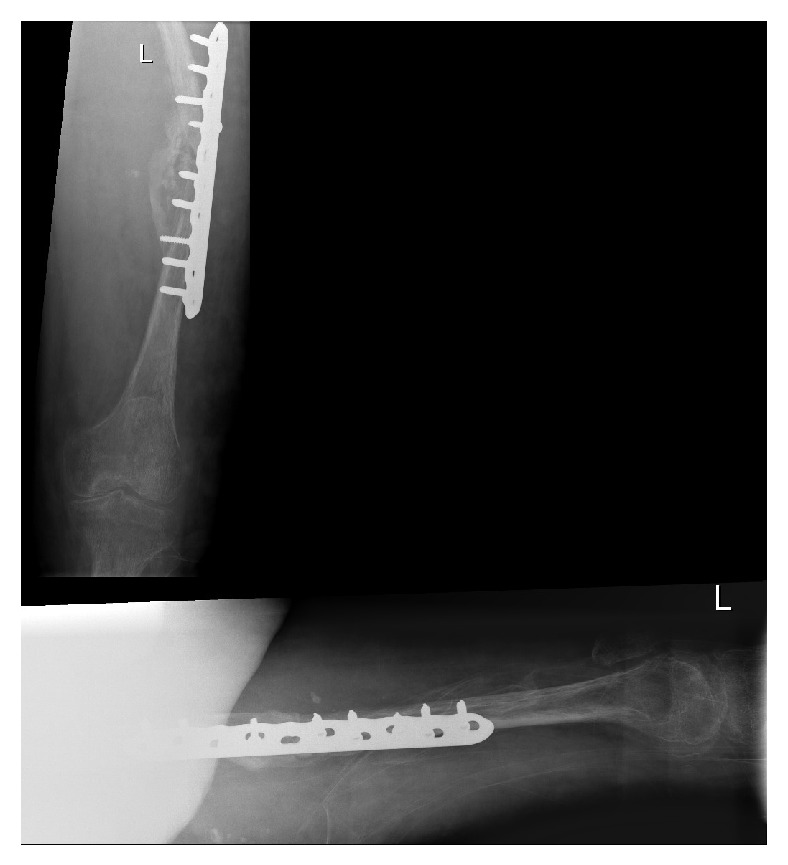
AP and lateral radiograph of the left femur after 10 weeks of LIPUS therapy.
